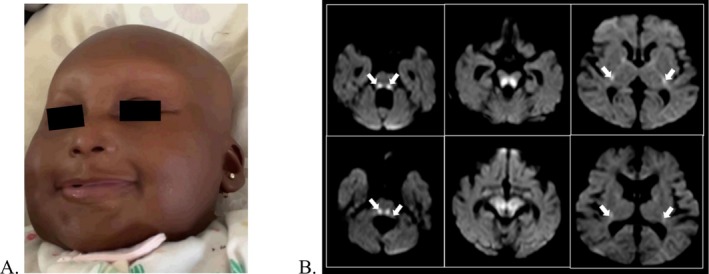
Investigations of our patient. (A) Clinical photograph of the patient in this report, demonstrating distinctive craniofacial features. Consent for publication was obtained from the patient's legal guardian at time of manuscript production. (B) MRI Brain demonstrating T2 hyperintensity along the bilateral dorsal pons and midbrain, cerebellar peduncles, and internal capsules, corresponding with concerns for diffusion restriction. These findings can be suggestive of a metabolic derangement affecting deep white matter tracts and brainstem structures.
